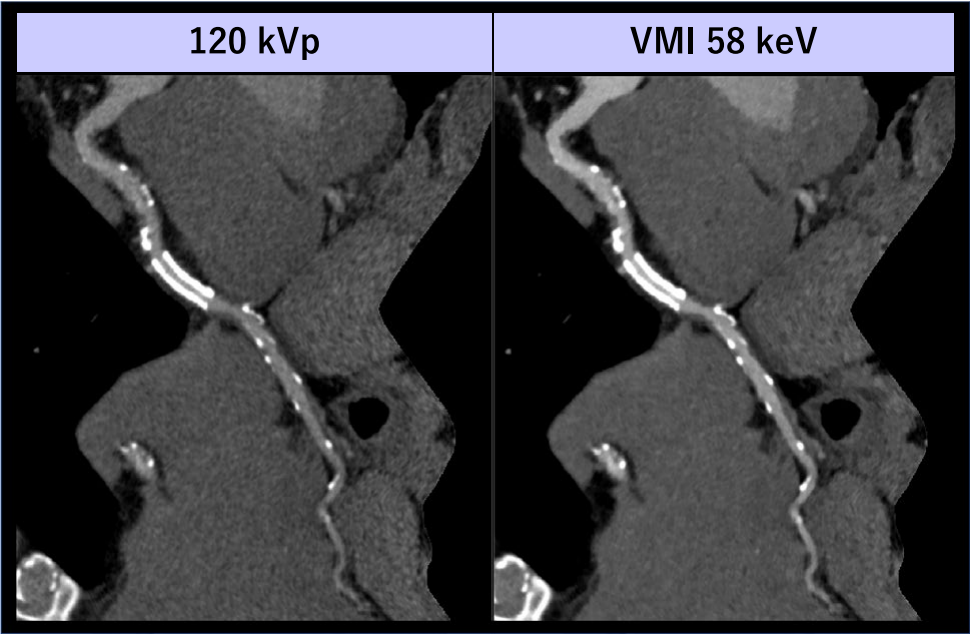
A 70-year-old man (BMI 27 kg/m^2^) post-PCI underwent coronary CTA using dual-layer detector scanner with a low dose of ICM (21 mL, 350 mgI/mL). The low keV VMI provided sufficient coronary enhancement while preserving the visibility of in-stent patency. Image courtesy of Minamino Cardiovascular Hospital
